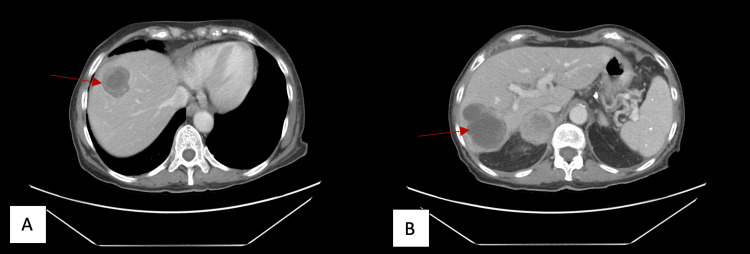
Axial CT abdomen and pelvis portal venous phase shows two hypoattenuating liver masses with rim enhancement and internal septations measuring 4.9 cm x 4.1 cm (A) and 6.7 cm x 5.6 cm (B) in the superior and inferior right hepatic lobes, respectively
